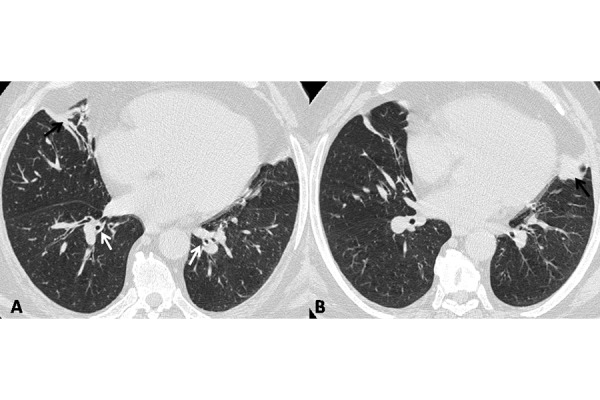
Axial view of HRCT shows the peribronchial thickening (white arrows) and peripheral areas of parenchymal consolidation (black arrows) into the middle lobe and lingula.
